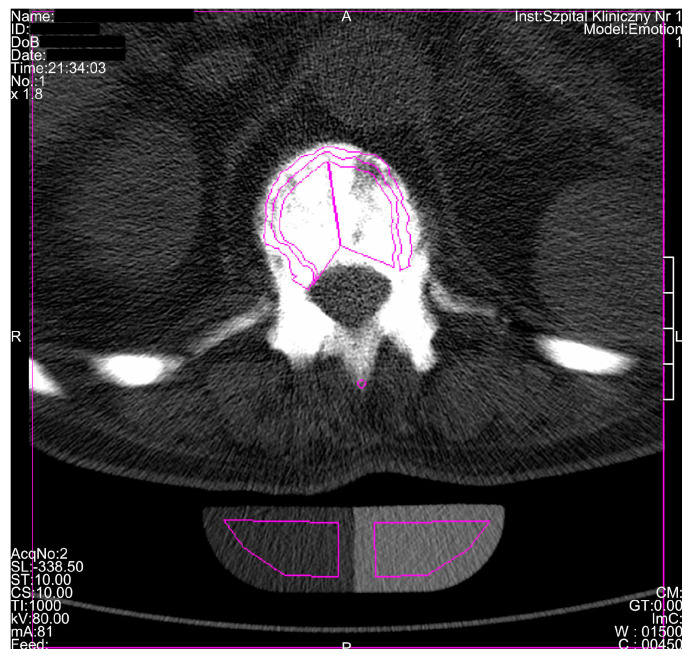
Volumetric bone mineral density (vBMD) measurement in the trabecular and cortical bone compartments of Th12 vertebrae using quantitative computed tomography (QCT) method and Osteo CT application package (Software Version B10/2004A), and expressed quantitatively as calcium hydroxyapatite (Ca-HA) density in the trabecular (TbCa-HA) and cortical bone (CbCa-HA). CT: the self-name of the software.
